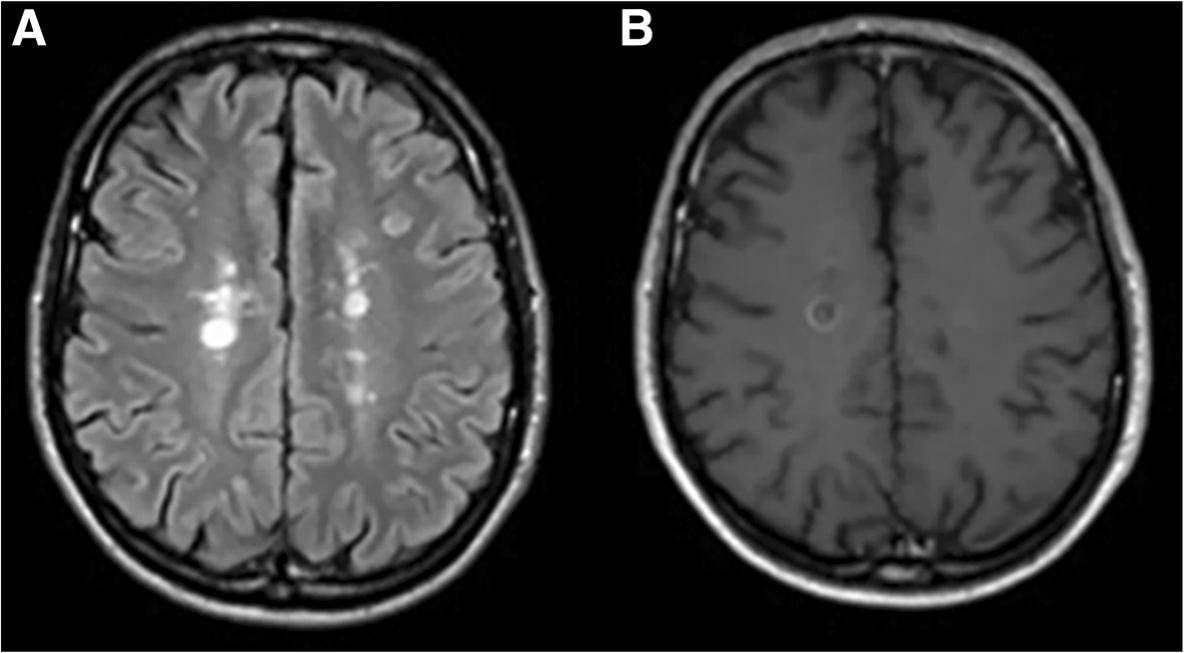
Magnetic resonance imaging (MRI) scan in a 35-year-old woman with relapsing-remitting multiple sclerosis (MS) imaged at 36 h after lumbar puncture showing bilateral multiple and partially confluent hyperintense lesions in periventricular white matter on fluid-attenuated inversion recovery (FLAIR) axial images (a). The ring enhancement characterizing the lesion located in the left hemisphere, at the level of centrum semiovale, was recognized on contrast-enhanced T1-weighted axial spin echo images (b) by one neuroradiologist, but not by the other. After consensus, this lesion was classified as Gadolium (Gd) enhanced and the patient as MRI active (Gd+). The consensus was needed for the same reasons in 5/60 (8.3%) patients included in the study
